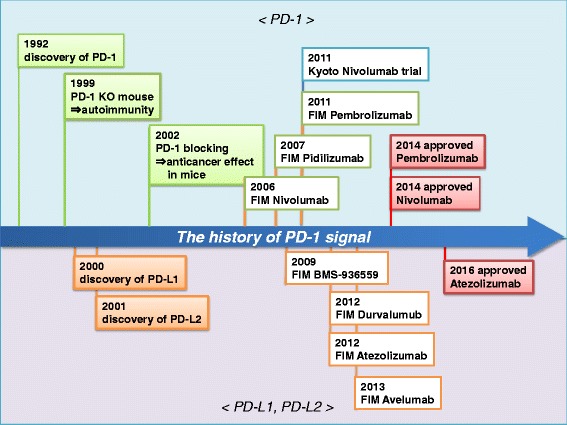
History of PD-1 research. Abbreviations: FIM, first in man; approved, FDA-approved; NCT, “National Clinical Trial” registry number in ClinicalTrials.gov in the United States; FIM Pembrolizumab (P07990/MK-3475-001/KEYNOTE-001), NCT01295827; FIM Pidilizumab (CT-011), NCT00532259; FIM BMS-936559 (MDX-1105), NCT00729664; FIM Atezolizumab, NCT01693562; FIM Durvalumab (MEDI4736), NCT01693562; FIM Avelumab, NCT01772004
